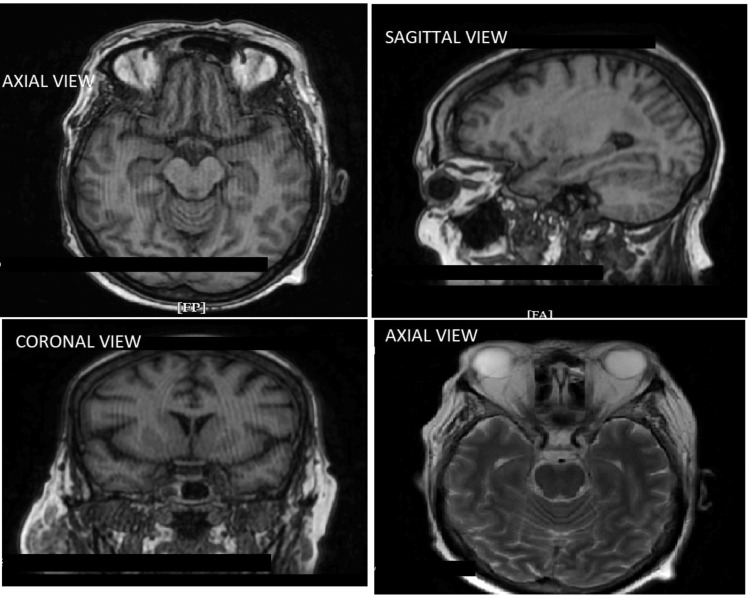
Non-contrast magnetic resonance imaging of the head showing no intracranial abnormalities
